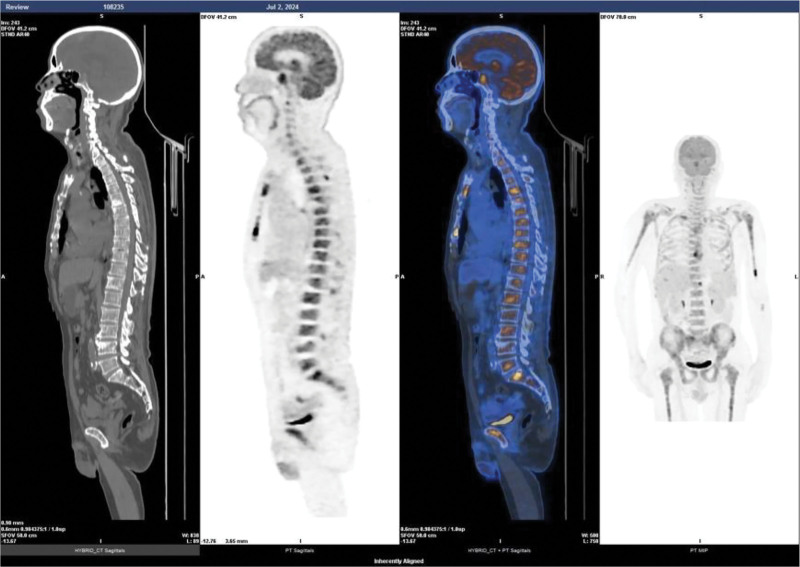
Whole body PET-CT revealing multiple areas of radiotracer uptake, indicating extensive bone destruction. PET-CT = positron emission tomography–computed tomography.
